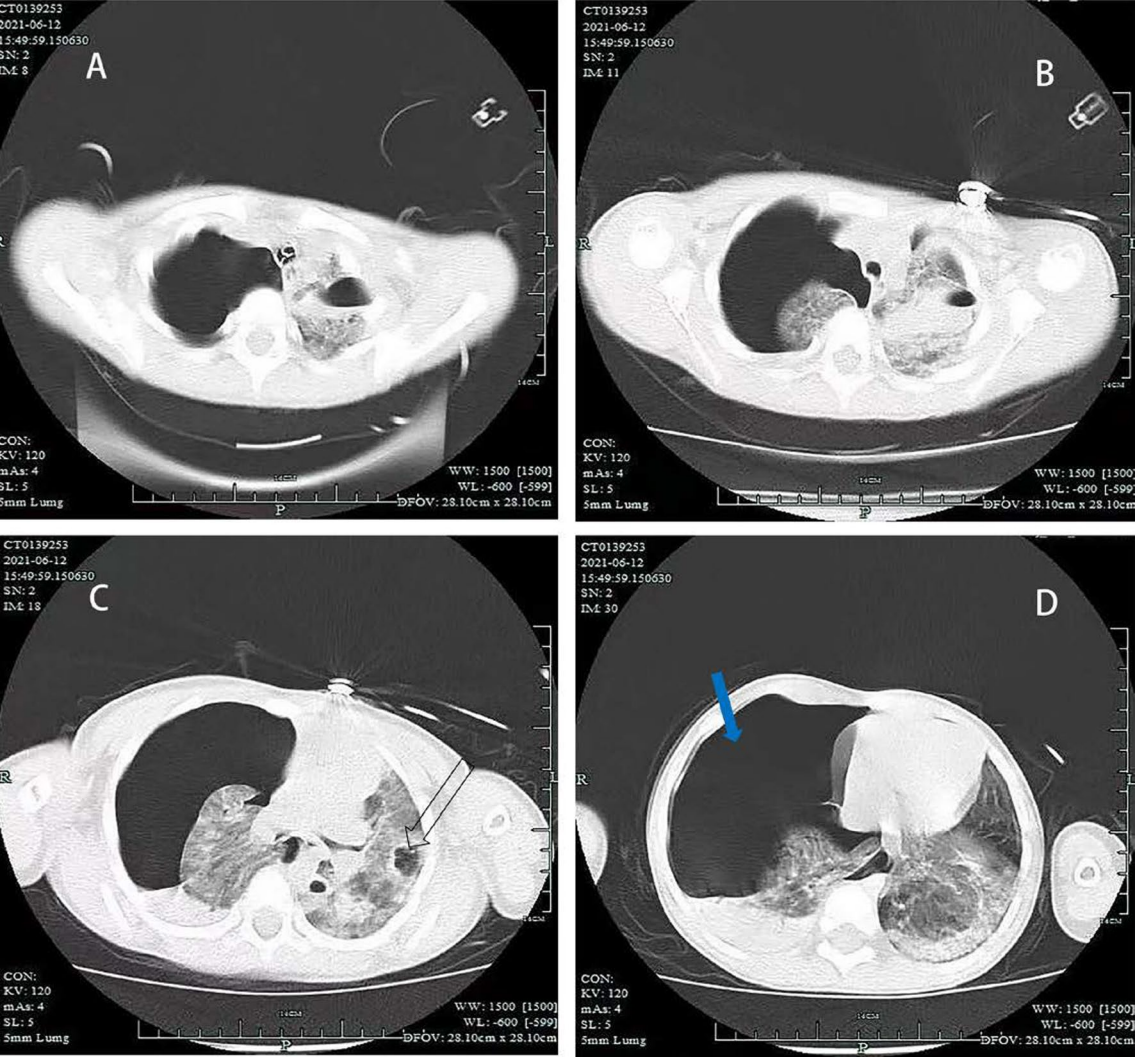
Chest CT (6.12) showed right pneumothorax and right lung compression 90% (blue arrow) and left traumatic wet lung (white arrow)
